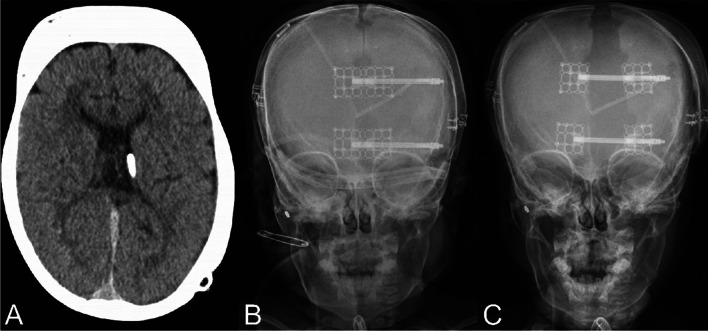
Patient with shunted hydrocephalus a preoperatively (computed tomography (CT) scan), b following distractor placement (frontal cephalogram), and c prior to distractor removal (frontal cephalogram) for posterior vault distraction osteogenesis (PVDO) with transverse distraction
